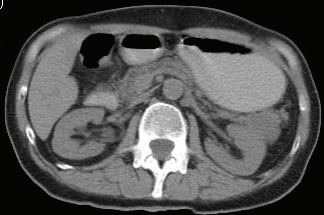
CT aspect of a pancreatic tail tumour
